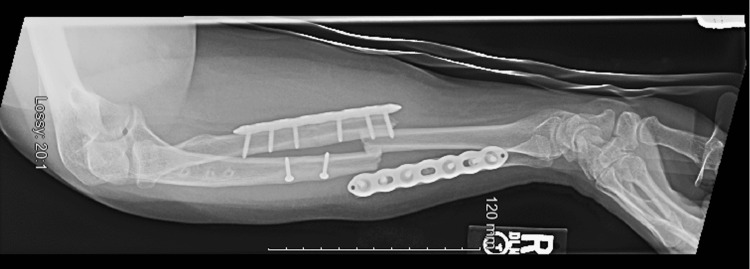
Lateral forearm radiograph at the time of the injury.
